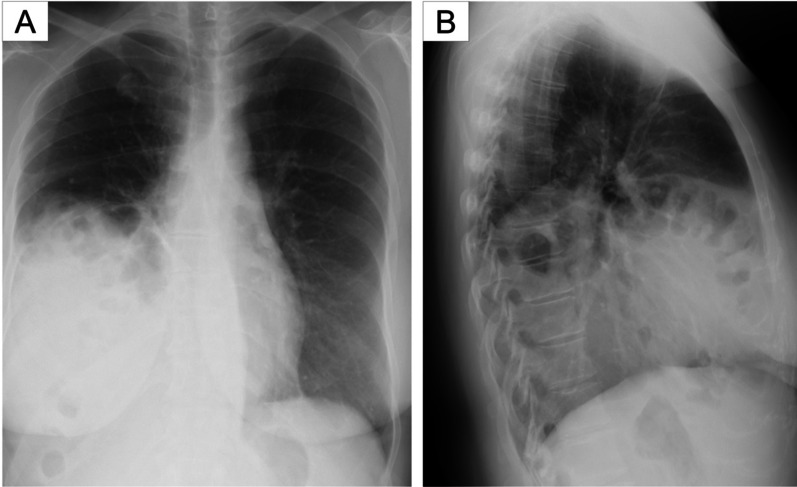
Preoperative chest radiography. a Frontal chest radiography showed a massive area of opacity in the right lung field. b Lateral chest radiography showed intestinal gas in opacity in the lung fields
